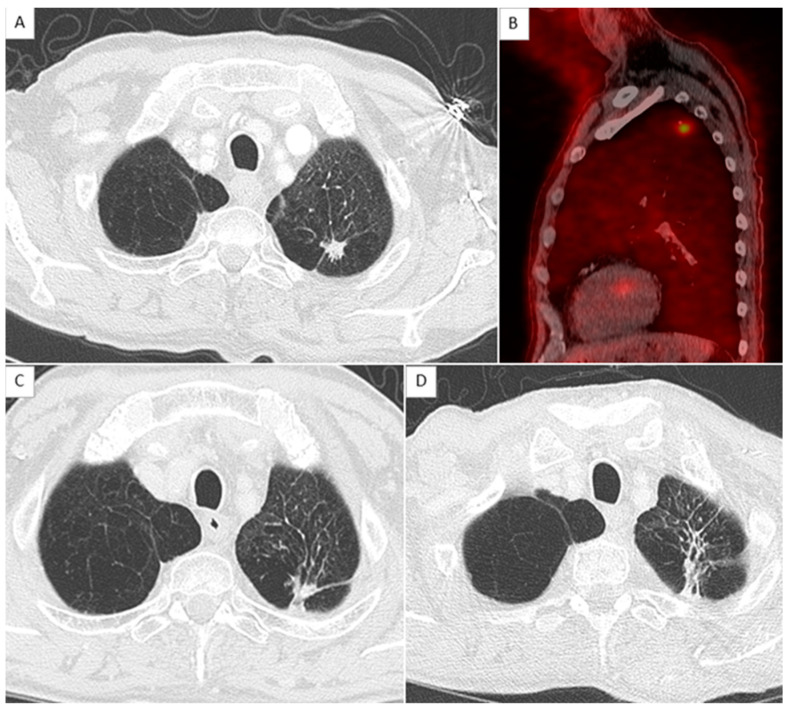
Example of the absence of local relapse with score at 0. (A,C,D) show axial chest CT in parenchymal window with an 11 mm nodule in the left apex corresponding to an adenocarcinoma, before, 1 year, and 2 years after stereotactic radiation therapy, respectively. (B) shows a sagittal reconstruction of merge PET and CT with a hypermetabolism of the nodule measured as SUV_max_ = 4.2.
